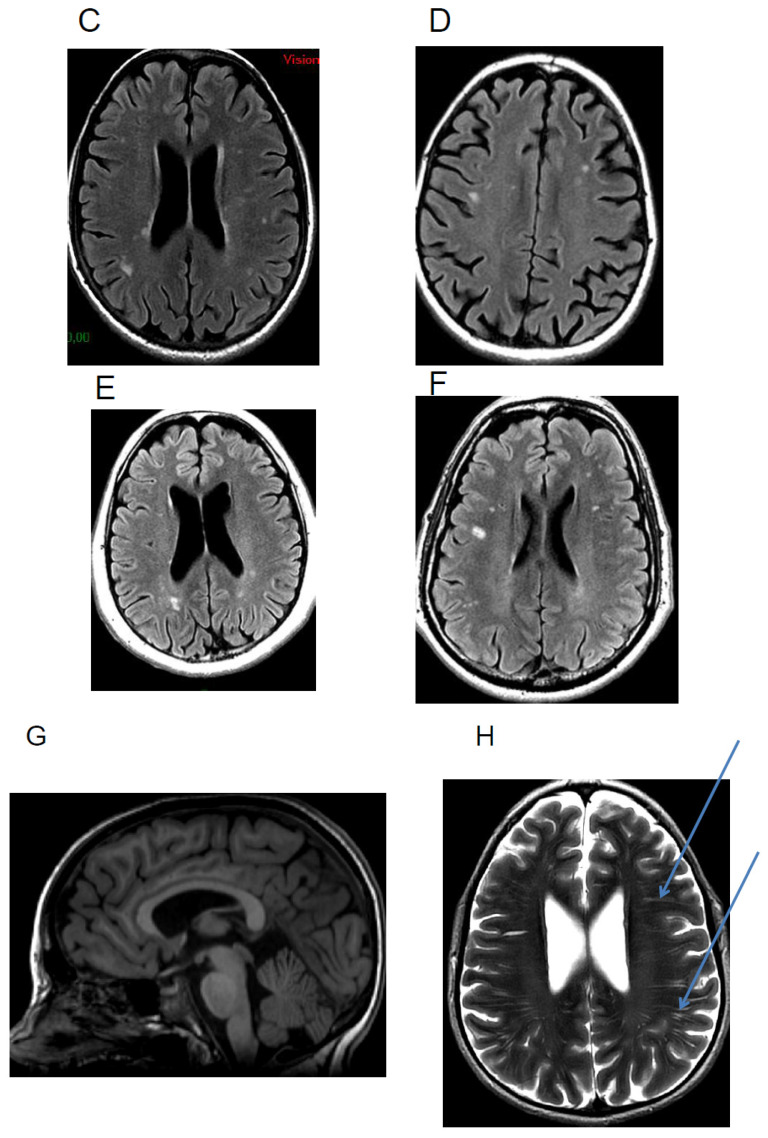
Axial FLAIR sequences. Examples of brain MRI anatomical images of cystinosis patients showing (A) an isolated ventricular dilatation (red arrow), (B) in another patient, cortical atrophy (green arrow) and diffuse white matter anomalies (blue arrow) associated with ventricular dilatation, (C–F) diffuse subcortical white matter hyperintensities, and (G,H) Wirshow or perivascular space enlargement (blue arrow)(images from N. Boddaert, Necker hospital).
